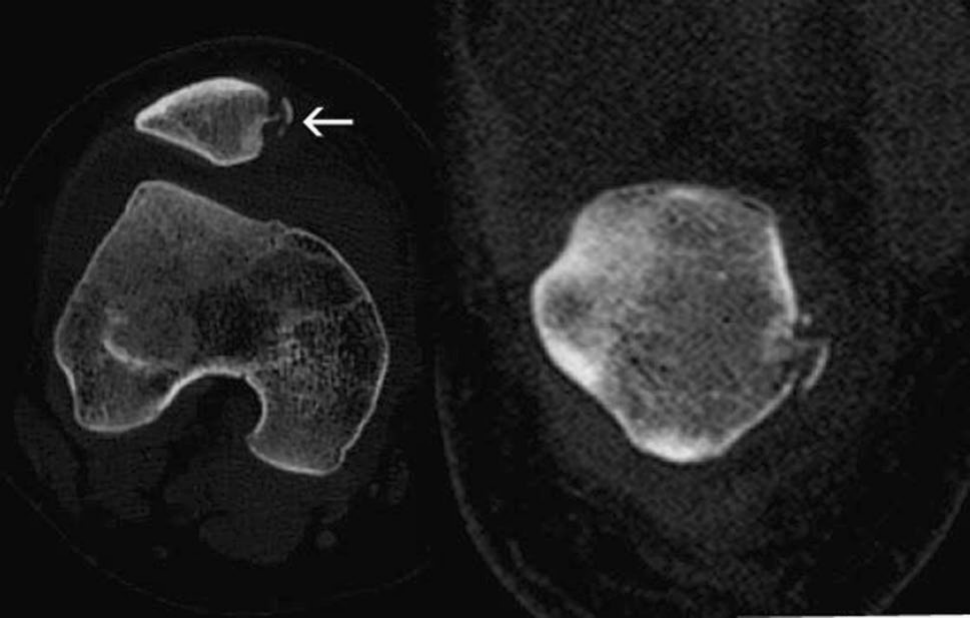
CT of dislocated right patella demonstrates lateral translation of the patella out of the trochlear notch, as well as the comminuted fracture of its medial aspect (arrow). The frontal image (on right) establishes that the location of the avulsion is not superomedial, but rather in the inferior half of the medial patella, mitigating against medial patellofemoral ligament involvement
